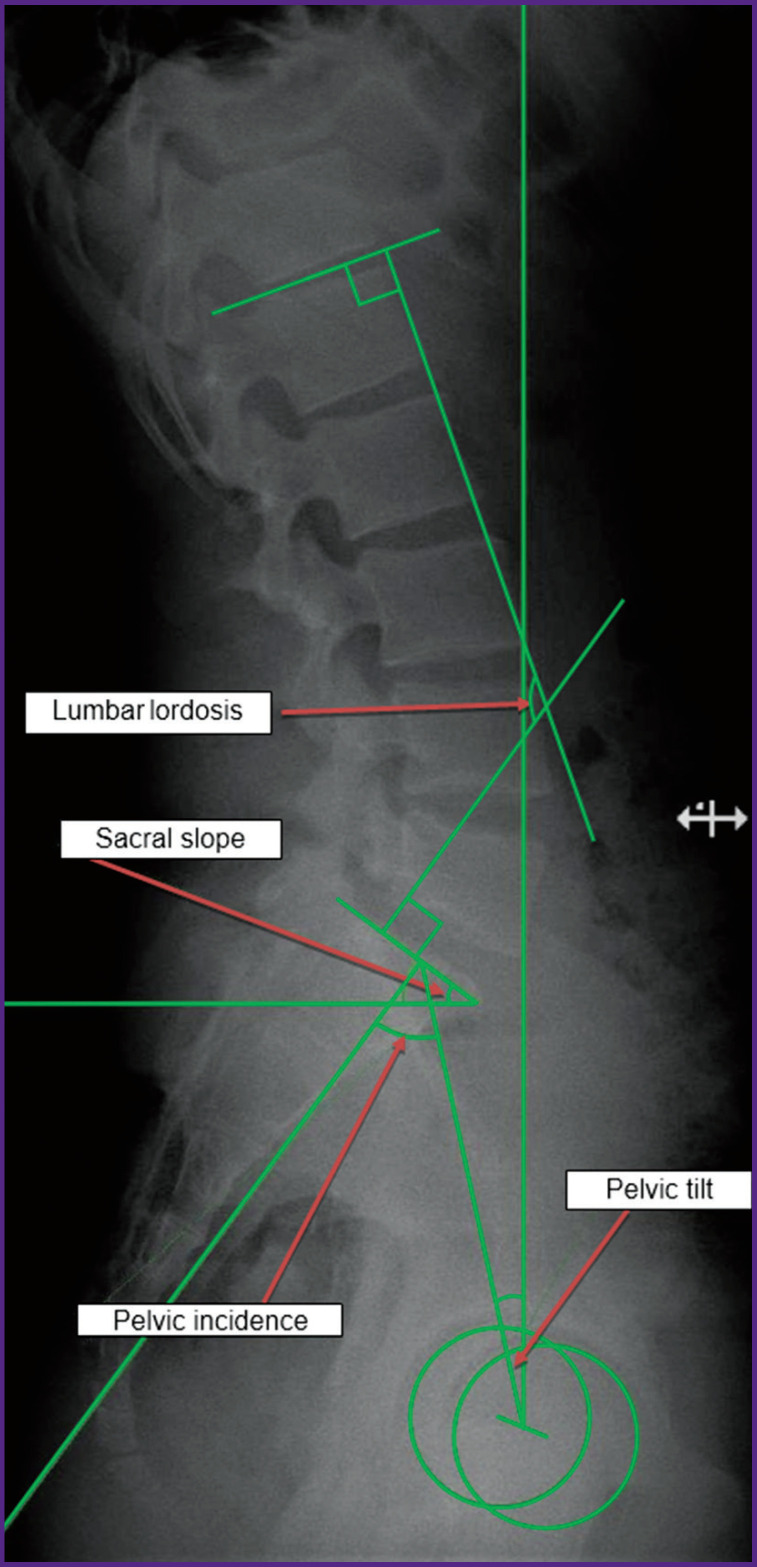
Angles of spinopelvic interrelationship (pelvic incidence, pelvic tilt, sacral slope, lumbar lordosis) on the radiograph
